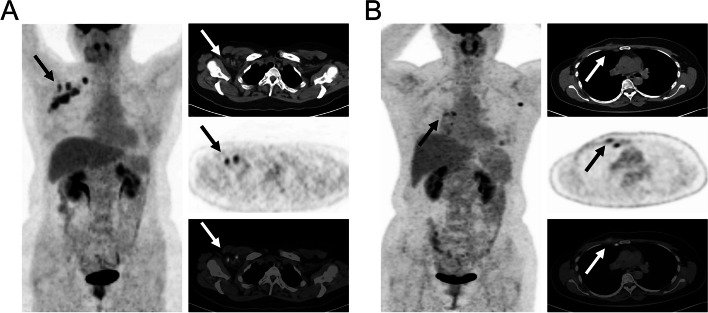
Representative images. A A 47‐year‐old female patient with MHBC underwent ^18^F‐FDG PET/CT scan, and the lesion of largest HI is showed by the arrow, the SUVmax = 3.76, SUVmean = 2.48, HI_50%_ = 1.516, and her PFS has not reached within 20.47 months of follow-up; B A 51‐year‐old female patient with MHBC underwent ^18^F‐FDG PET/CT scan, the lesion of largest HI_50%_ is showed by the arrow, the SUVmax = 4.83, SUVmean = 3.06, HI = 1.578, and her PFS was 3.09 months
